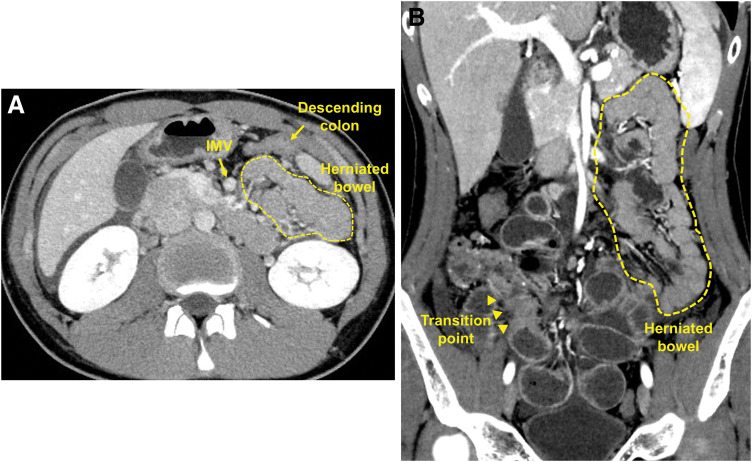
CT findings at the time of readmission. (A) Axial view. A segment of the small intestine was observed herniating into Landzert’s fossa (area enclosed by dashed lines and labeled “Herniated bowel”). The IMV coursing ventrally to the herniated loops and descending colon is indicated by arrows. (B) Coronal view. The concurrent recurrence of the left paraduodenal hernia is depicted (area enclosed by dashed lines and labeled “Herniated bowel”). The transition point in the terminal ileum, which represents the twisted adhesion and the primary cause of recurrent obstruction, is also shown (indicated by arrowheads). Note that marked bowel dilation within the hernia sac is not prominent, consistent with the clinical finding that the primary obstruction was at the terminal ileum. IMV, inferior mesenteric vein
